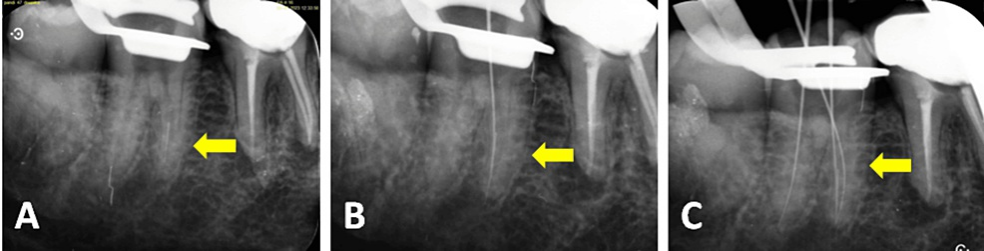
Intraoral periapical radiographs of 47 (A) A 15 K file separated in the apical third of the mesiolingual canal, (B) separated file bypassed using a 15 K file, and (C) working length determination
